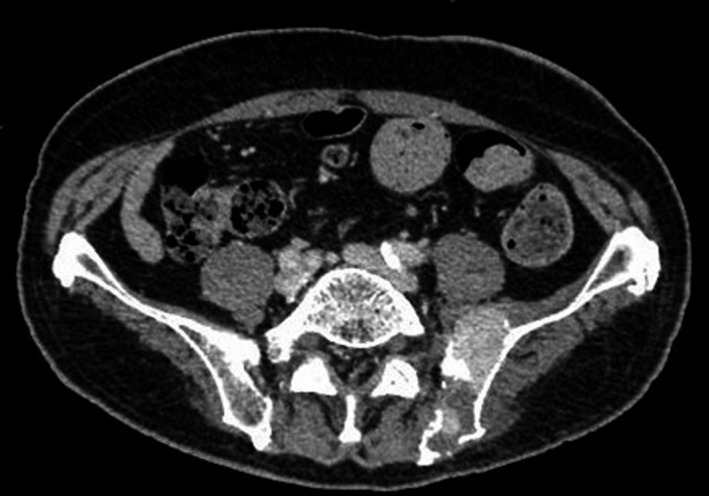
Abdominal computed tomography showed a left iliac tumor
